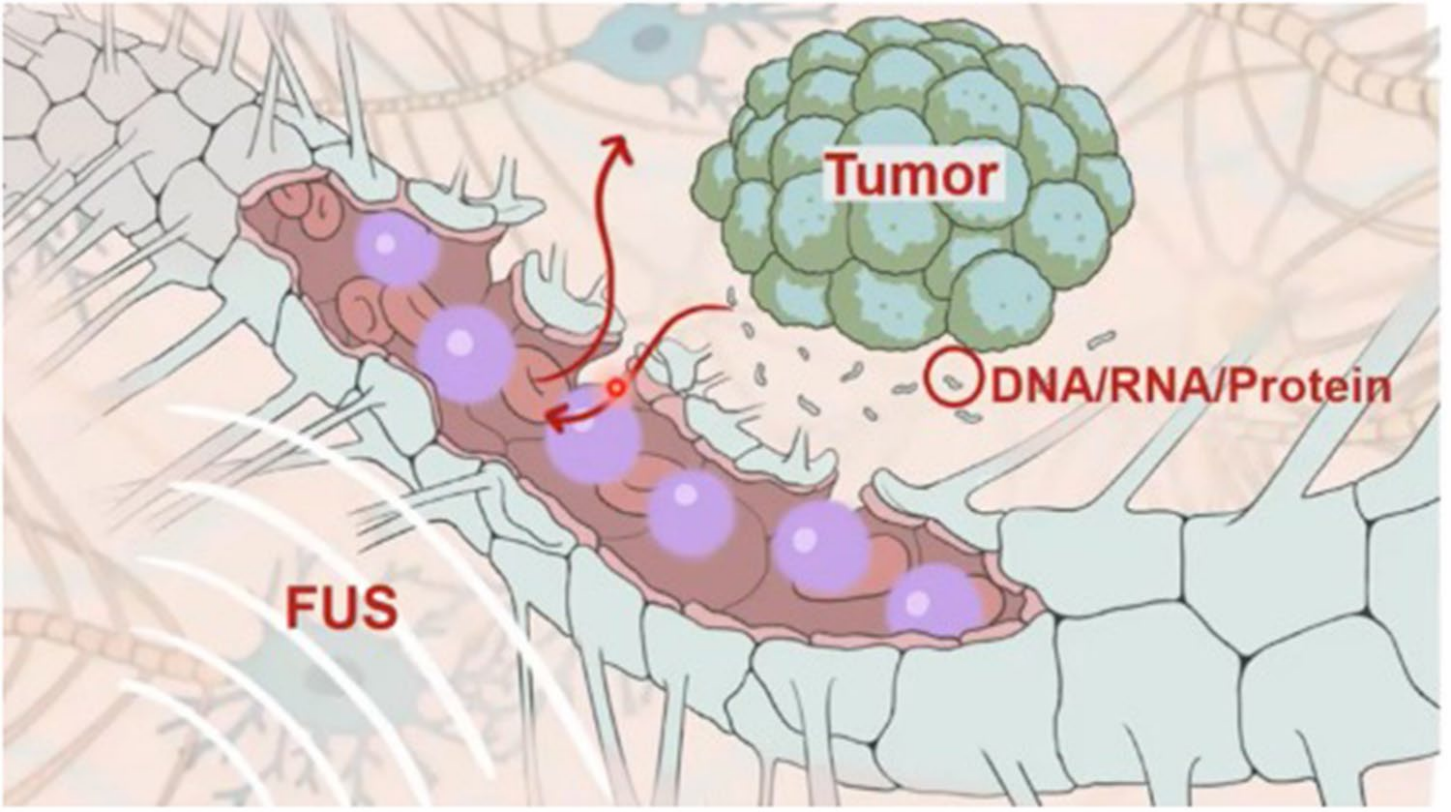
Blood–Brain Barrier Opening for Liquid Biopsy. Blood–brain barrier opening with focused ultrasound (FUS) allows bidirectional flow of molecules. DNA, RNA, and protein tumor markers enter the peripheral circulation, where they can be collected and analyzed. Unpublished image courtesy of Hong Chen, PhD, Washington University in St. Louis
